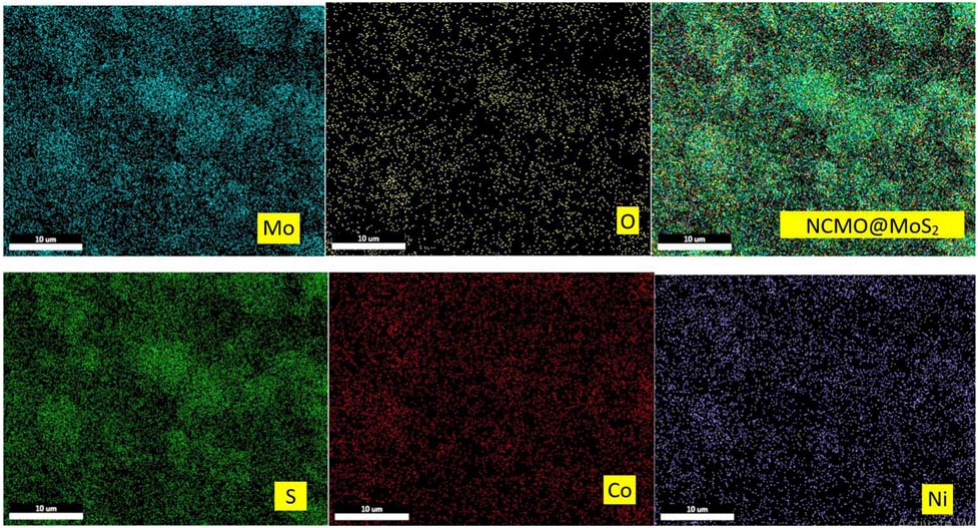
X-ray mapping analysis of NCMO@MoS_2_.
